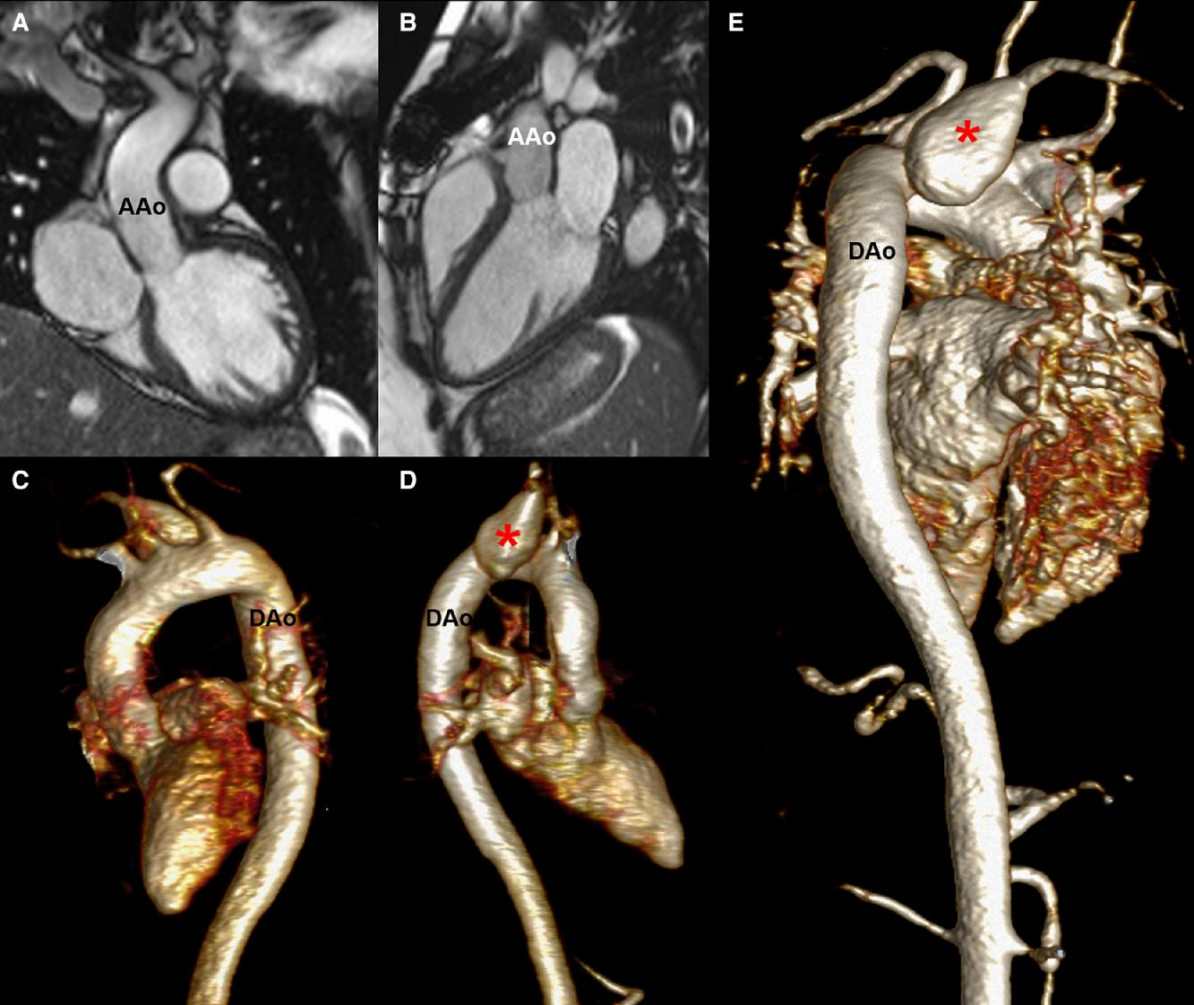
Cardiovascular magnetic resonance images after delivery. Left ventricular outflow tract and three-chamber views demonstrating the replaced ascending aorta (*A*, *B*). Three-dimensional reconstructions (*C–E*) derived from contrast-enhanced magnetic resonance angiography showing the thoracic aorta and the subclavian artery aneurysm (star). AAo, ascending aorta; Dao, descending aorta; LV, left ventricle.
